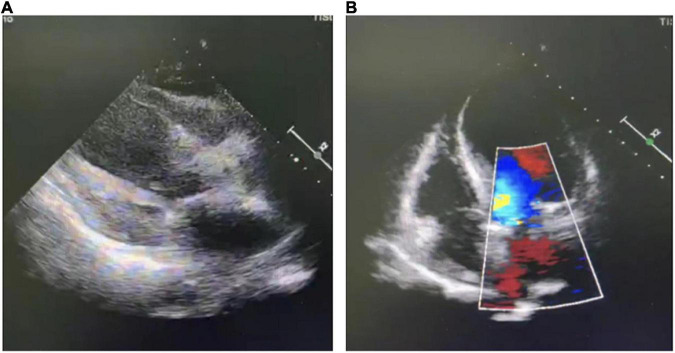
Ultrasonic echocardiogram. (A) Parasternal long axis view; (B) apical four chambers view.
